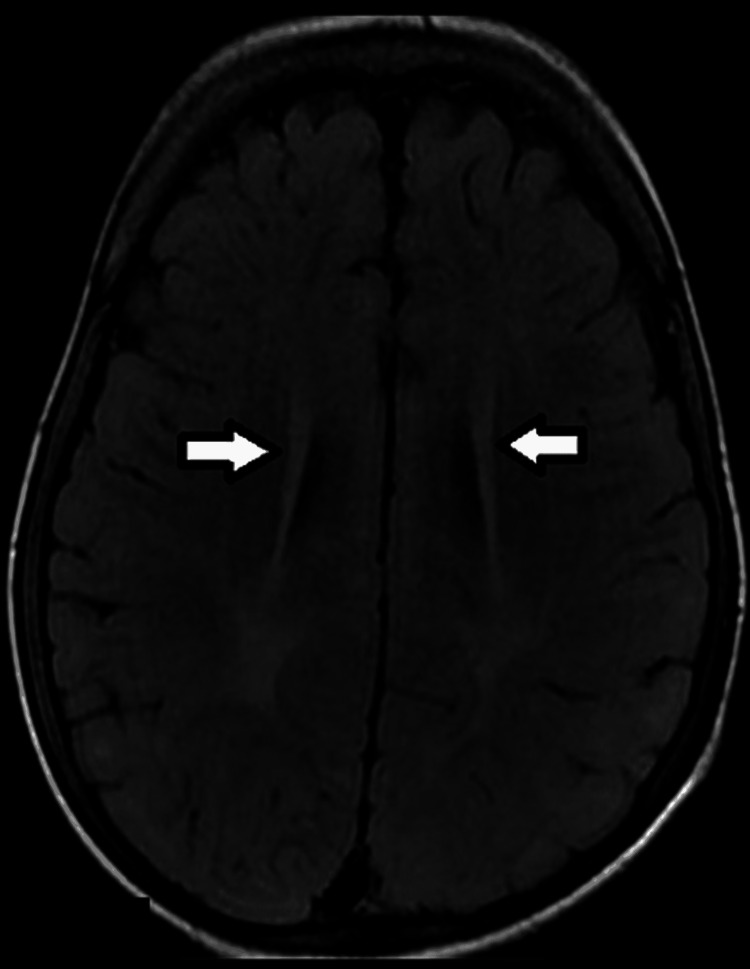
Axial T2 FLAIR image in a 18-month male showing an abnormal hyperintense signal in bilateral periventricular region, consistent with changes of periventricular leukomalacia (white arrows).
